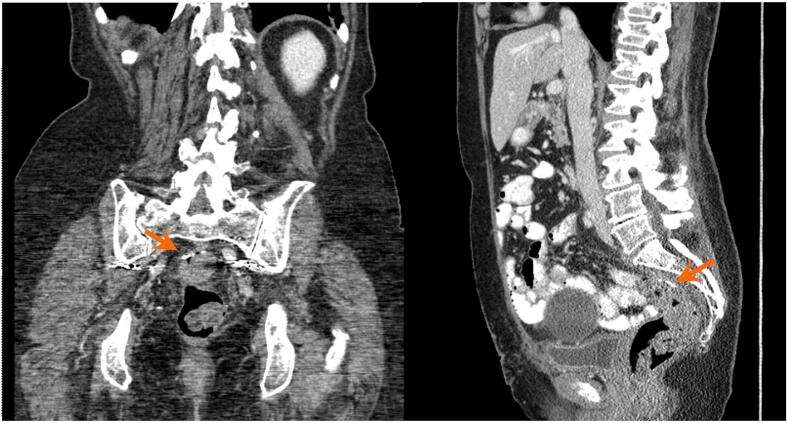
CT approximately 7 months after treatment shows complete resolution of the collection previously seen posterior to the suture line (arrows).
